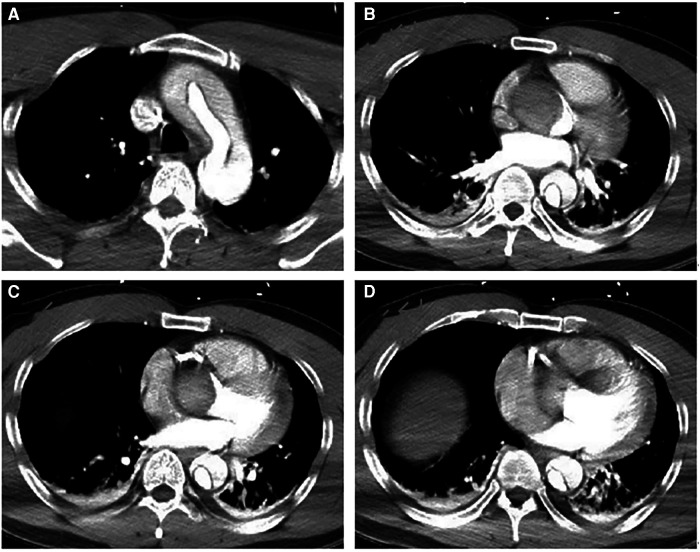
Preoperative aortic CTA image. (A–D) CTA shows the false lumen of the ascending aorta compressing the true lumen, extending above the opening of the right coronary artery. They indicate a thick hematoma in the false lumen compressing the right coronary artery.
